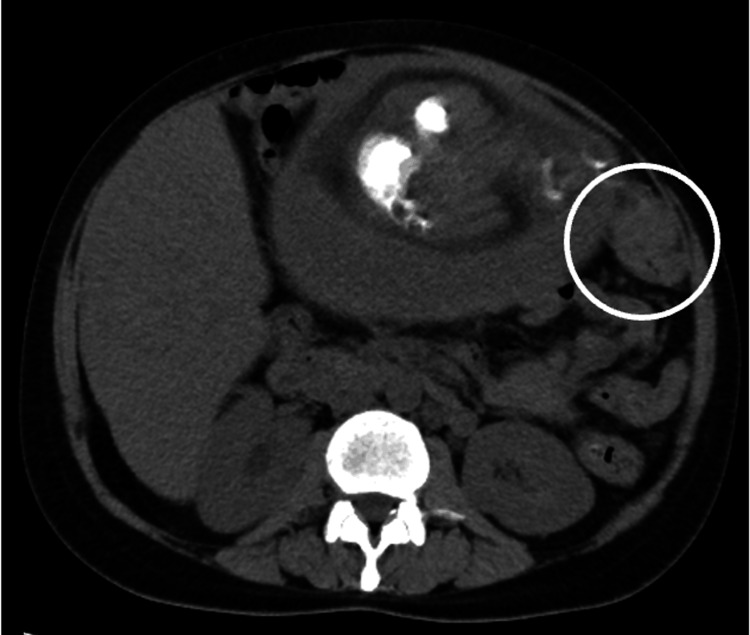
Non-contrast computed tomography (CT) revealed a suspected lesion in the transverse colon (◯)
